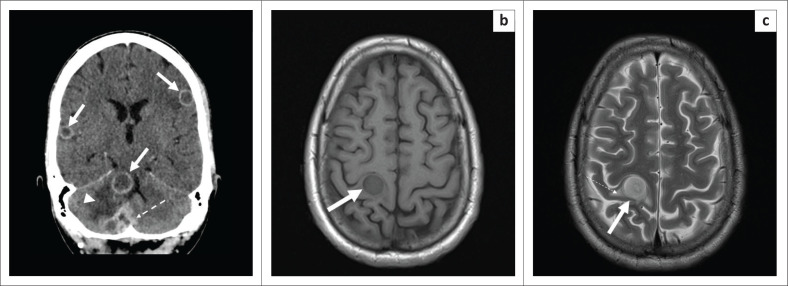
A 28-year-old female with disseminated tuberculosis who presented with a 3-month history of weakness and horizontal nystagmus. (a) Axial post-contrast computed tomography brain through the cerebellum reveals several hypodense supra- and infra-tentorial rim-enhancing tuberculomas (white solid arrows) of which some are clustered (white arrow with dashed line) with surrounding oedema (white arrow head). (b) Axial magnetic resonance imaging of the brain through the centrum semiovale of the same patient reveals a non-caseating granuloma, T1-weighted hypointense (white solid arrow) and (c) T2-weighted hyperintense (white solid arrow) with minimal surrounding oedema (white arrow with dashed line). Tuberculosis was confirmed on cerebrospinal fluid analysis.
